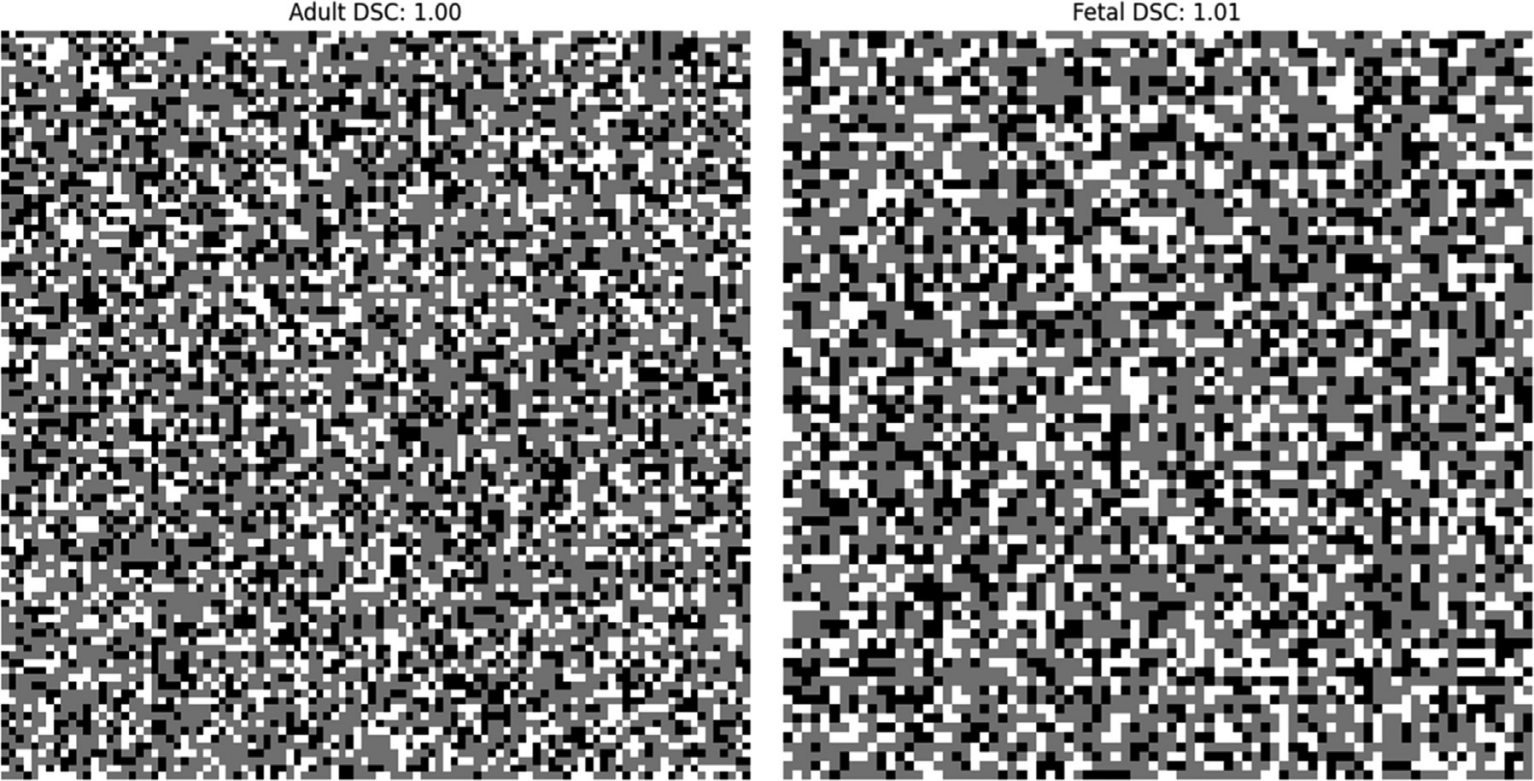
Anatomical Accuracy Evaluation Using Dice Similarity Coefficient for Adult and Fetal Echocardiography.
